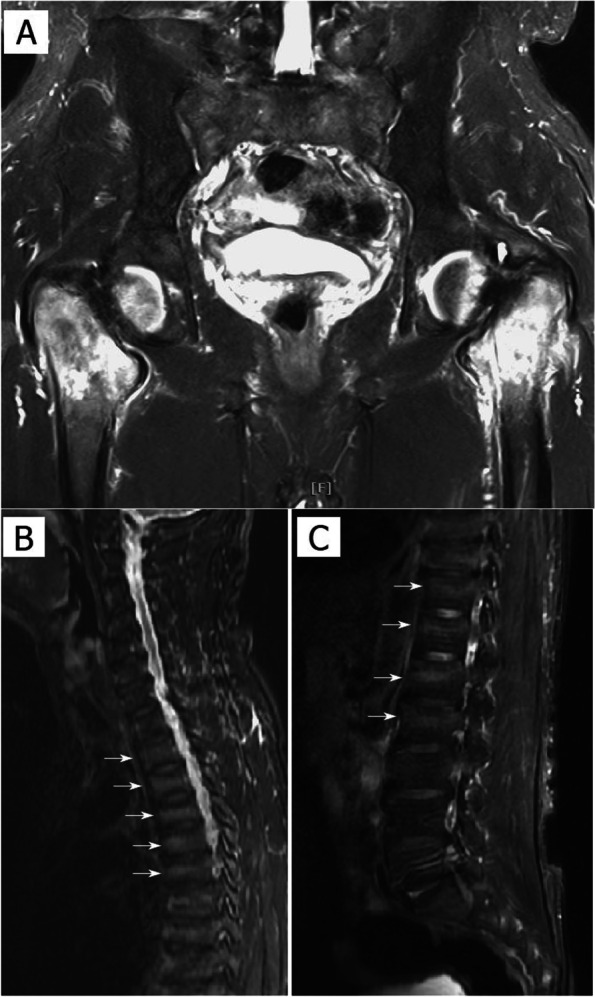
MRI of the pelvis and spine with STIR image. A High signal over the bilateral femoral neck; increased signal over the sacrum ala. B and C Multiple band-like lesions at the vert
